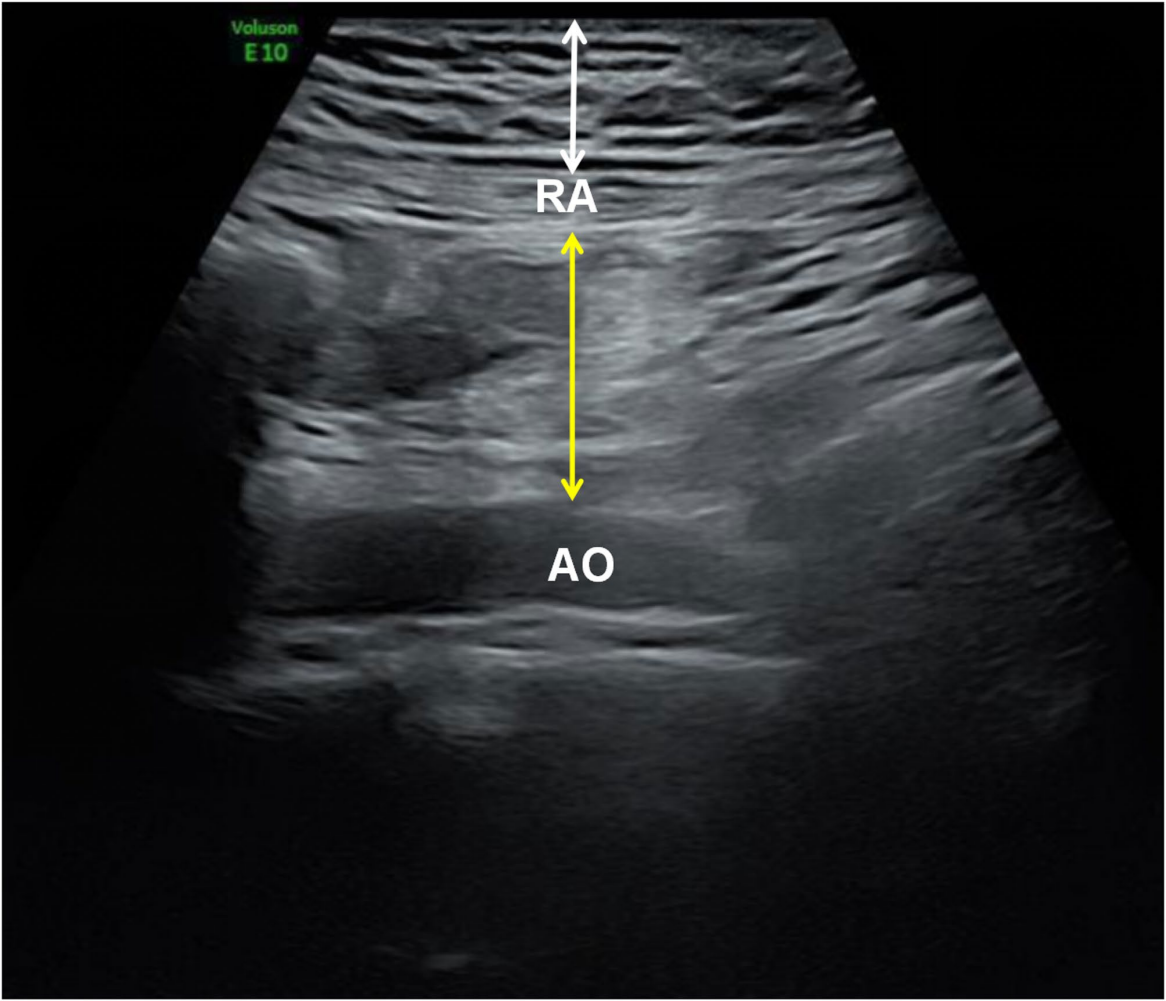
Ultrasound measurement of maternal abdominal SAT(white arrow) and VAT (yellow arrow) indicators. RA, rectus abdominis muscle; AO, abdominal aorta; SAT, subcutaneous adipose tissue; VAT, visceral adipose tissue.
